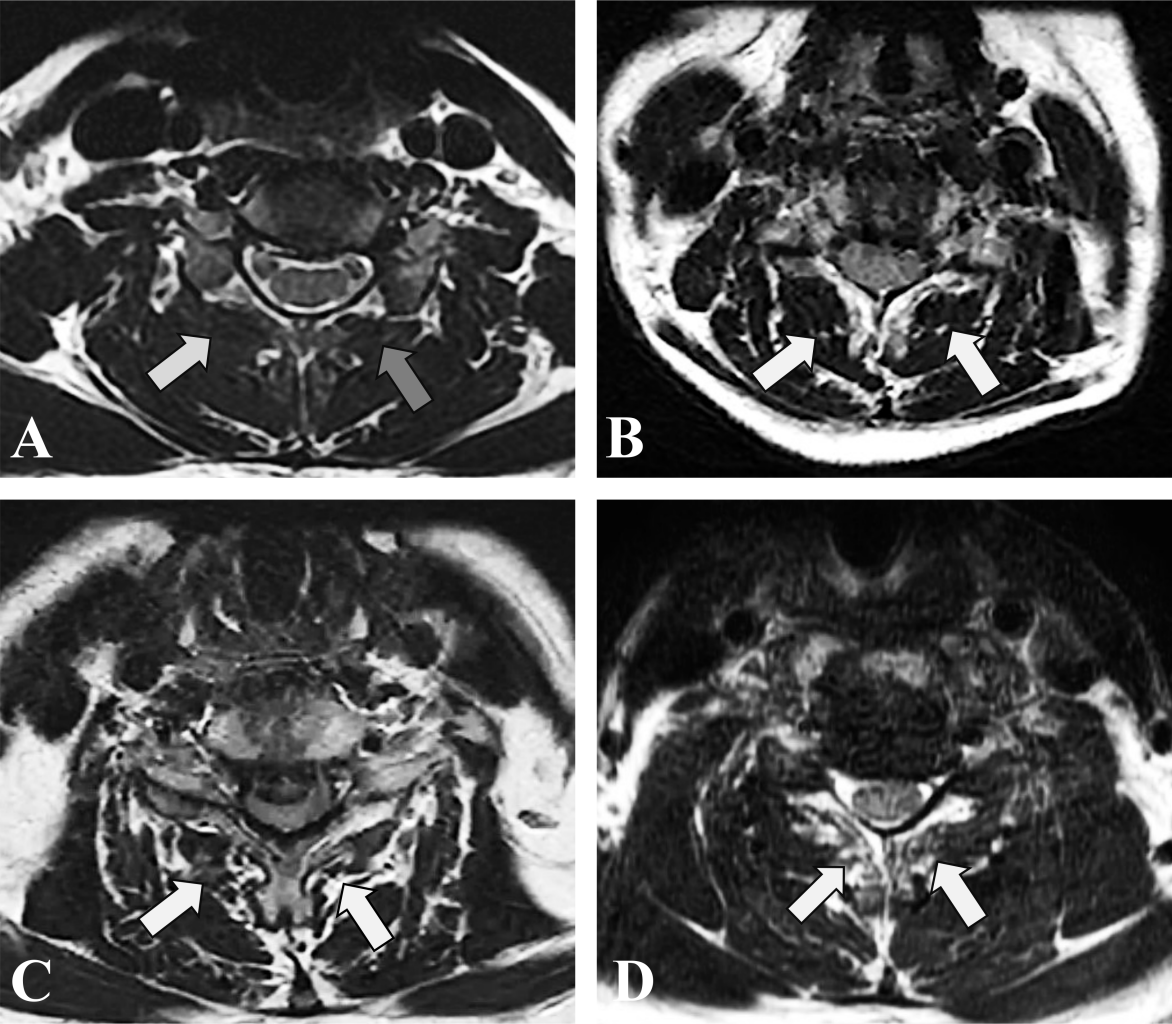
T2-weighted axial MRI section demonstrating fatty infiltration of muscle multifidus belly at C5/6. (A) Goutalier Grade 0 (white arrow), Goutalier Grade 1 (grey arrow); (B) Goutalier Grade 2. (C) Goutalier Grade 3. (D) Goutalier Grade 4.
